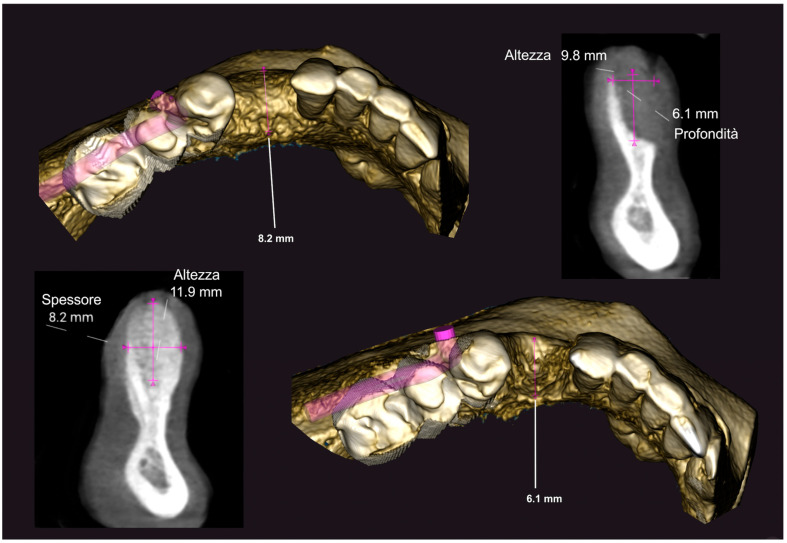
CBCT comparison, baseline and 8 months after GBR.
